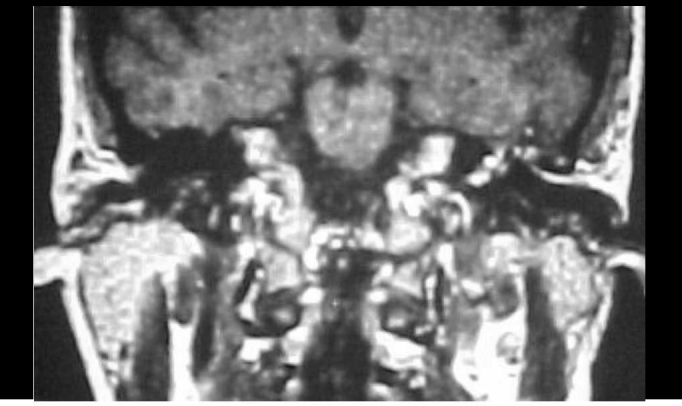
MRI of temporal bone using gadolinium contrast showing hypervascularized tissue in the left middle ear.
